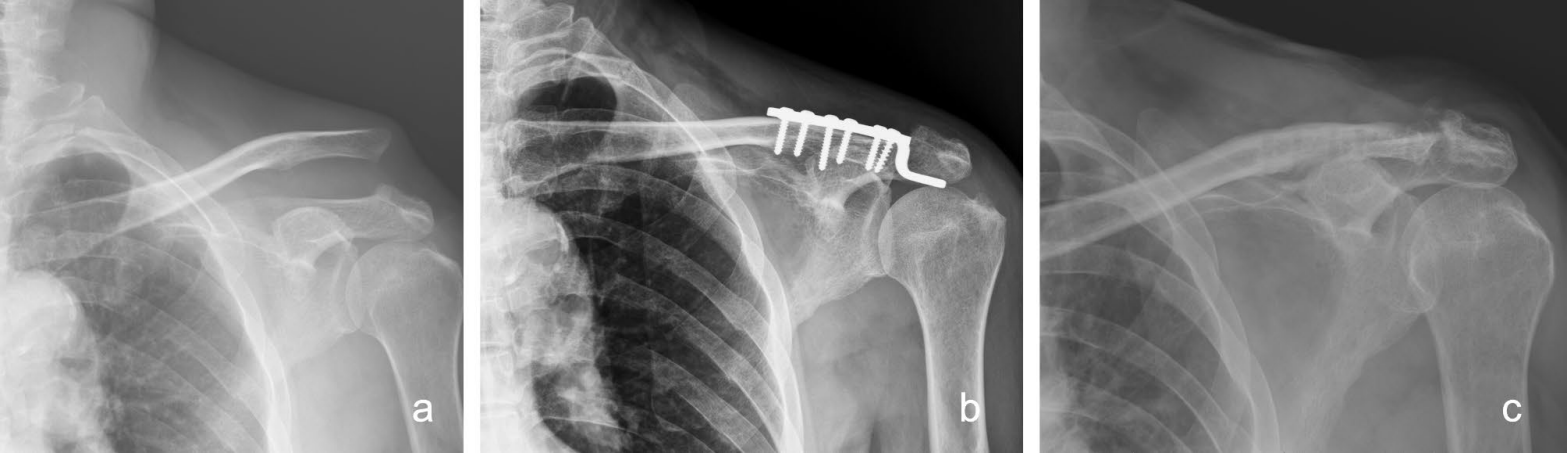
A 62 years old male presented with left shoulder acromion osteolysis after hook plate fixation. (a) Preoperative plain radiograph. (b) Status post open reduction and fixation with hook plate. (c) Acromion osteolysis noted at postoperative 1-year follow up
